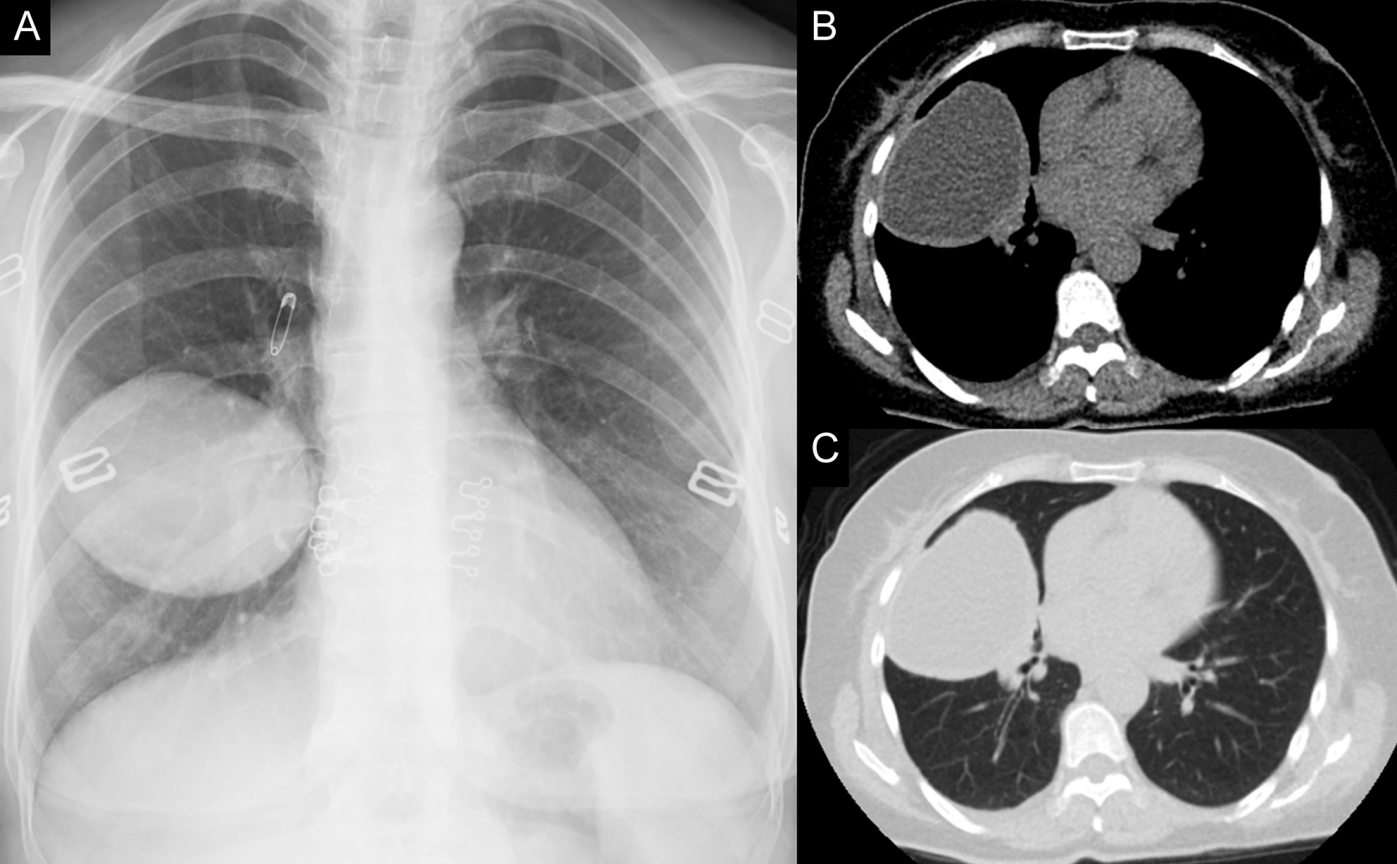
In a 60-year-old female, an intact hydatid cyst of approximately 9 cm in diameter originating from the lower lobe of the right lung is seen in posteroanterior chest x-ray (a), thorax computed tomography sections of the mediastinum (b) and parenchyma windows (c).
